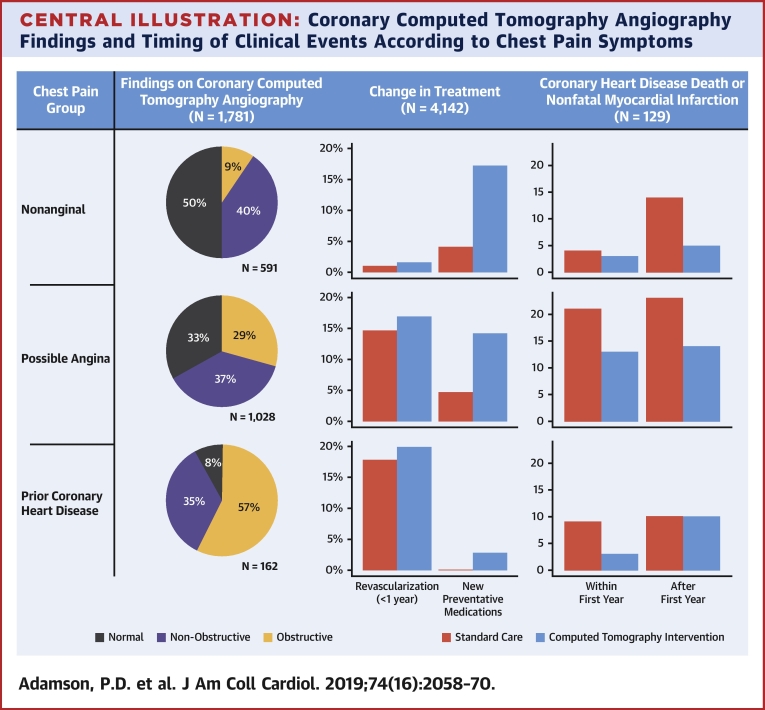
Coronary Computed Tomography Angiography Findings and Timing of Clinical Events According to Chest Pain Symptoms Findings on coronary computed tomography angiography (left), changes in provision of preventative medications and early coronary revascularization (center), and timing of coronary heart disease death or nonfatal myocardial infarction events (right) according to the National Institute of Health and Care Excellence guideline classification of chest pain symptoms.
